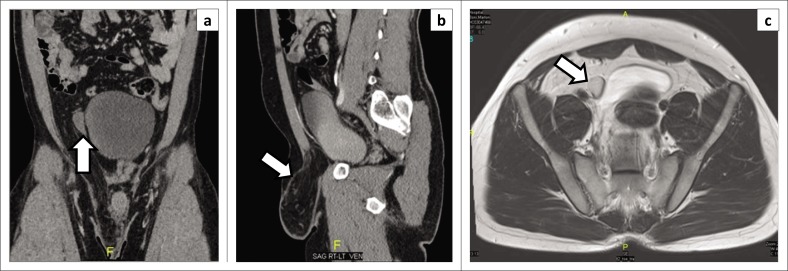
A 30-year-old male presented to the emergency department with pain in the right inguinal region with a large inguinoscrotal hernia on clinical examination: (a) Coronal and (b) sagittal images of a computed tomography abdomen demonstrated an undescended testis (arrow on image a) along its path in the right lower abdomen, located lateral to the surface of urinary bladder (U). There was an associated large right omental hernia (arrow on image b). (c) The undescended testis is hyperintense (arrow on image c) on axial T2-weighted magnetic resonance images.
